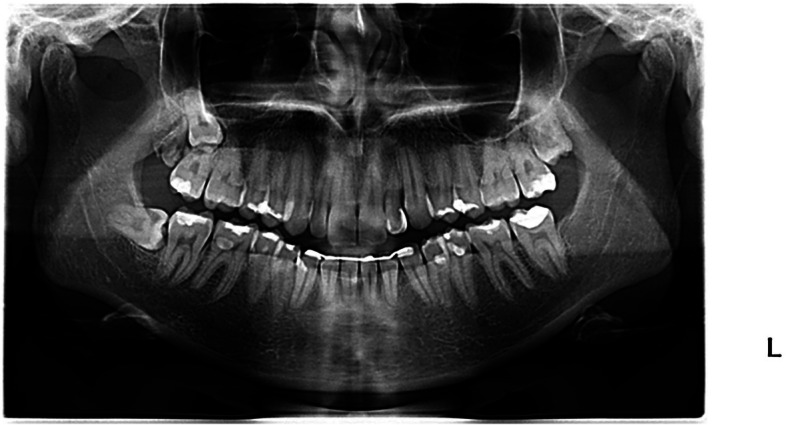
Panoramic radiograph number 9 used in the survey for evaluation of MTM 48
